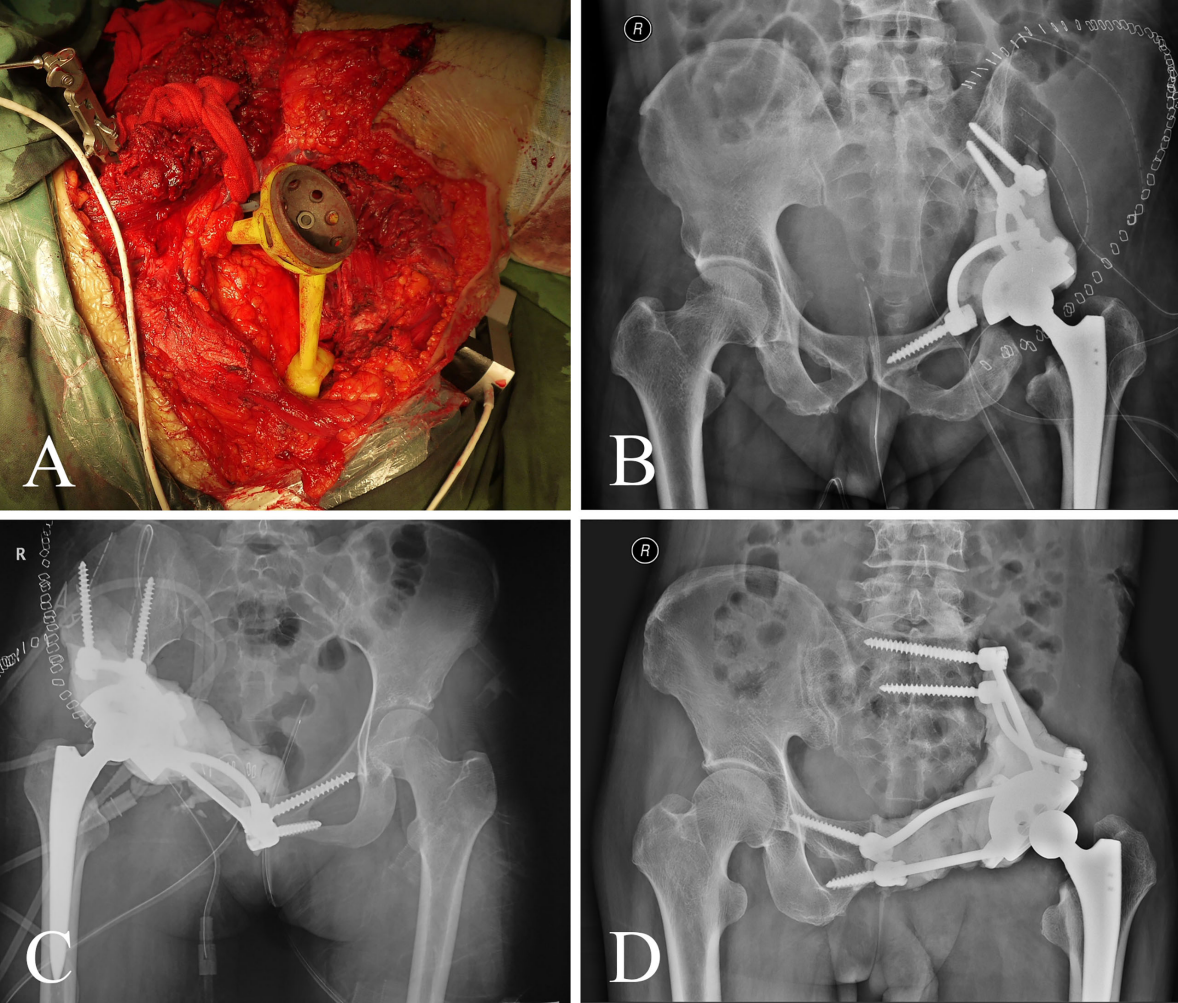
(A) The screw-rod-cage system was implanted after tumor excision. (B–D) Postoperative pelvic radiograph of screw-rod-cage system reconstruction.
